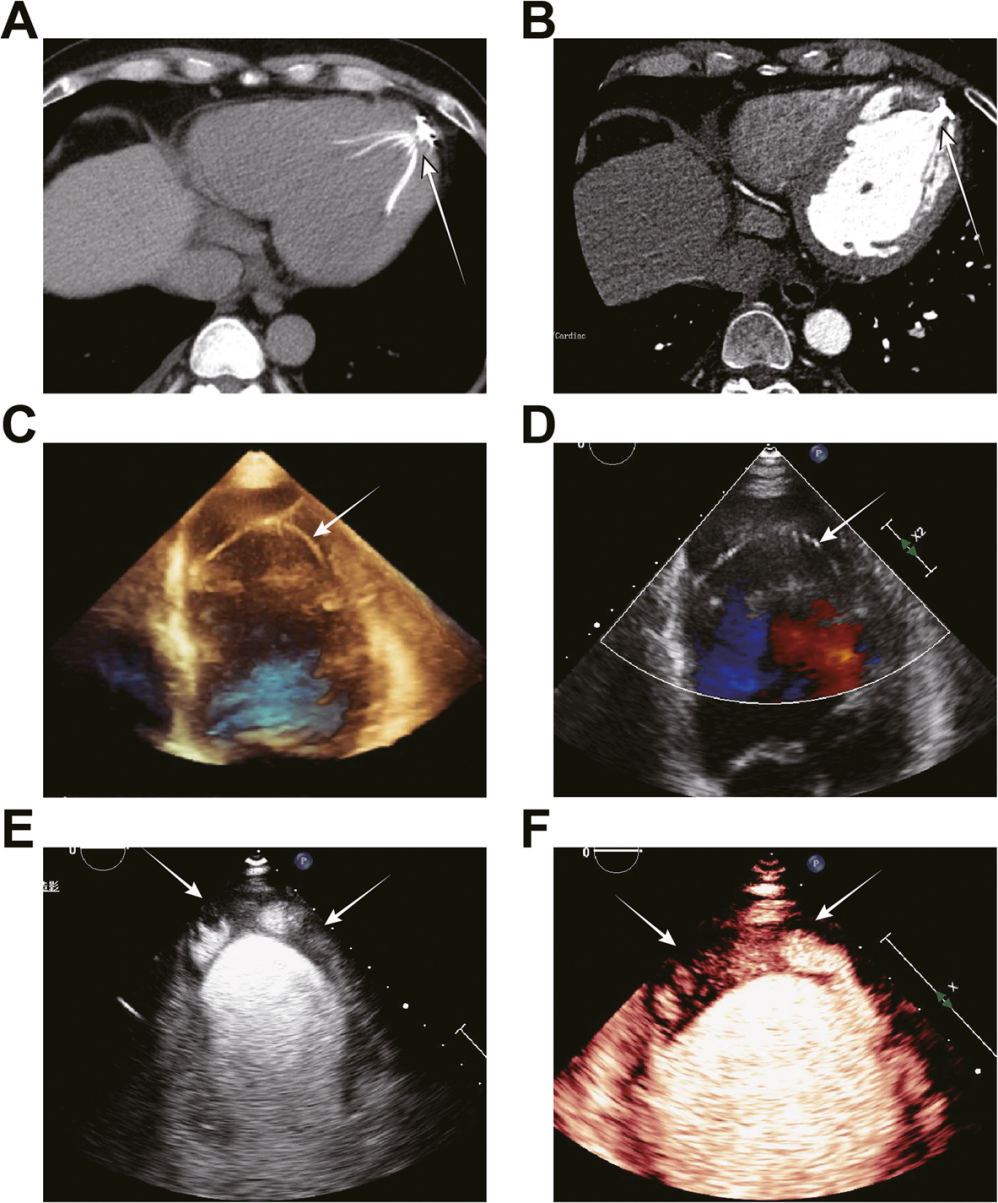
Case with left ventricular thrombus after device implantation by contrast echocardiography. a CT scan right after the PARACHUTE implantation to check potential device-related migration or embolization. b Latest follow-up detected by contrast-enhanced CT. The filling defect appeared in the apical left ventricle. c and d 3D-TTE and 2D-TEE were applied to exclude device migration, respectively. The white arrow indicates the implanted device. e and f Left ventricular thrombus confirmed by LVO and MCE, respectively. The white arrow points to the filling defect-implied LV apical thrombus
